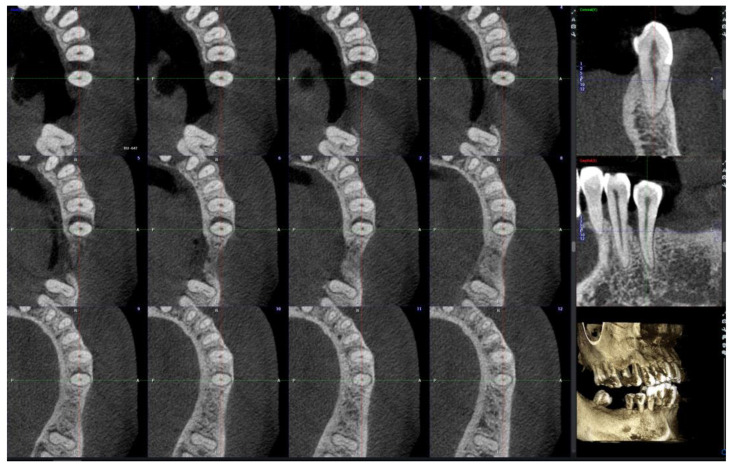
Example of CBCT slices in the coronal and sagittal views (right column), as well as axial slices at a distance of 0.5 mm apart, from the coronal (slice 1, upper left) to the apical direction (slice 12, lower right). The infrabony defect is visible on 7 axial slices (from slice 3 to 9).
